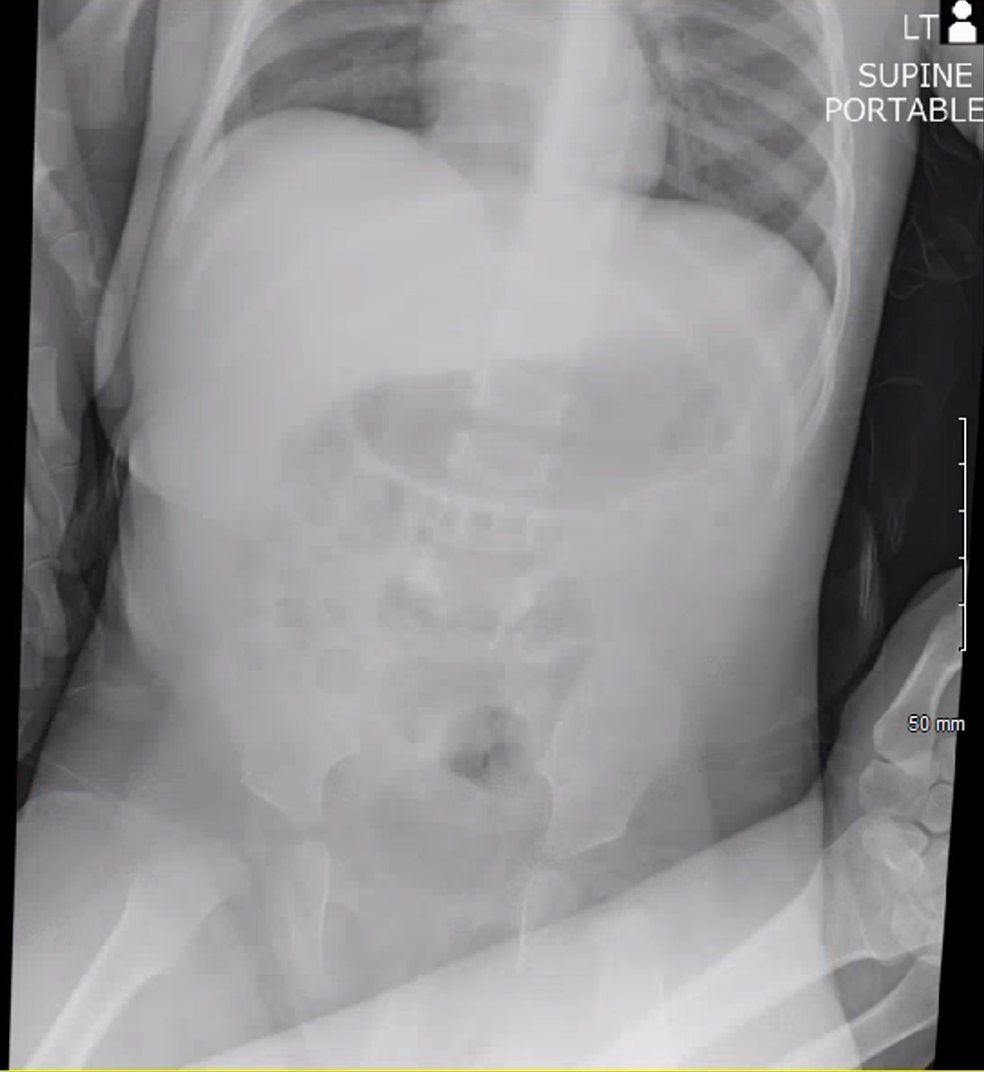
Abdominal X-ray reveals no evidence of ileus or obstruction.
